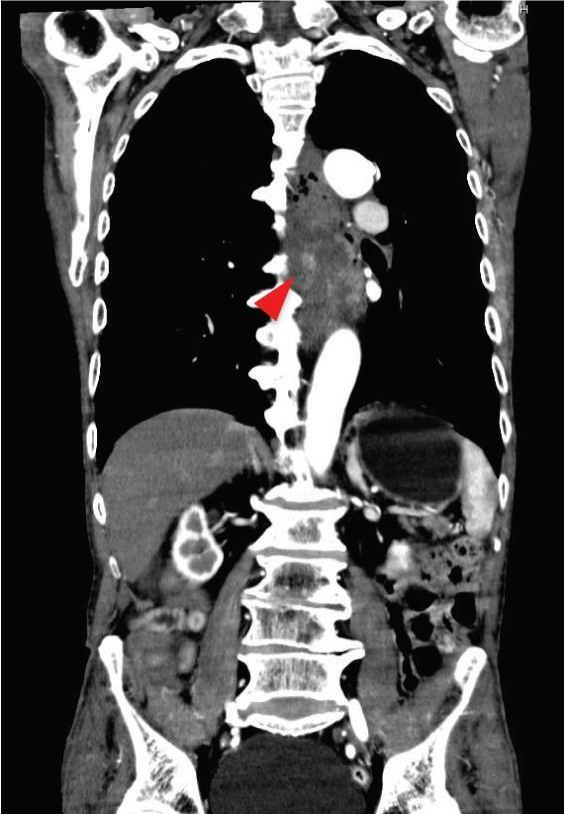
Enhanced computed tomography on arrival. Contrast-enhanced CT showed a large tumor in the middle to lower esophagus. Extravasation of contrast medium toward the esophageal lumen was observed (arrowhead).
